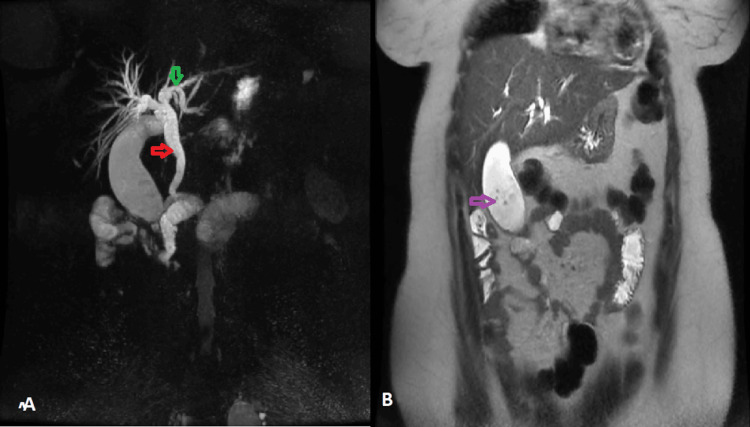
Magnetic resonance cholangiopancreatography A: 3D MRI showing dilated main bile duct at 9 mm (red arrow) and dilatation of intrahepatic bile ducts (green arrow), B: coronal slice in T2 showing gallstones (purple arrow) and absence of visualization of intrahepatic stones on MRI.
